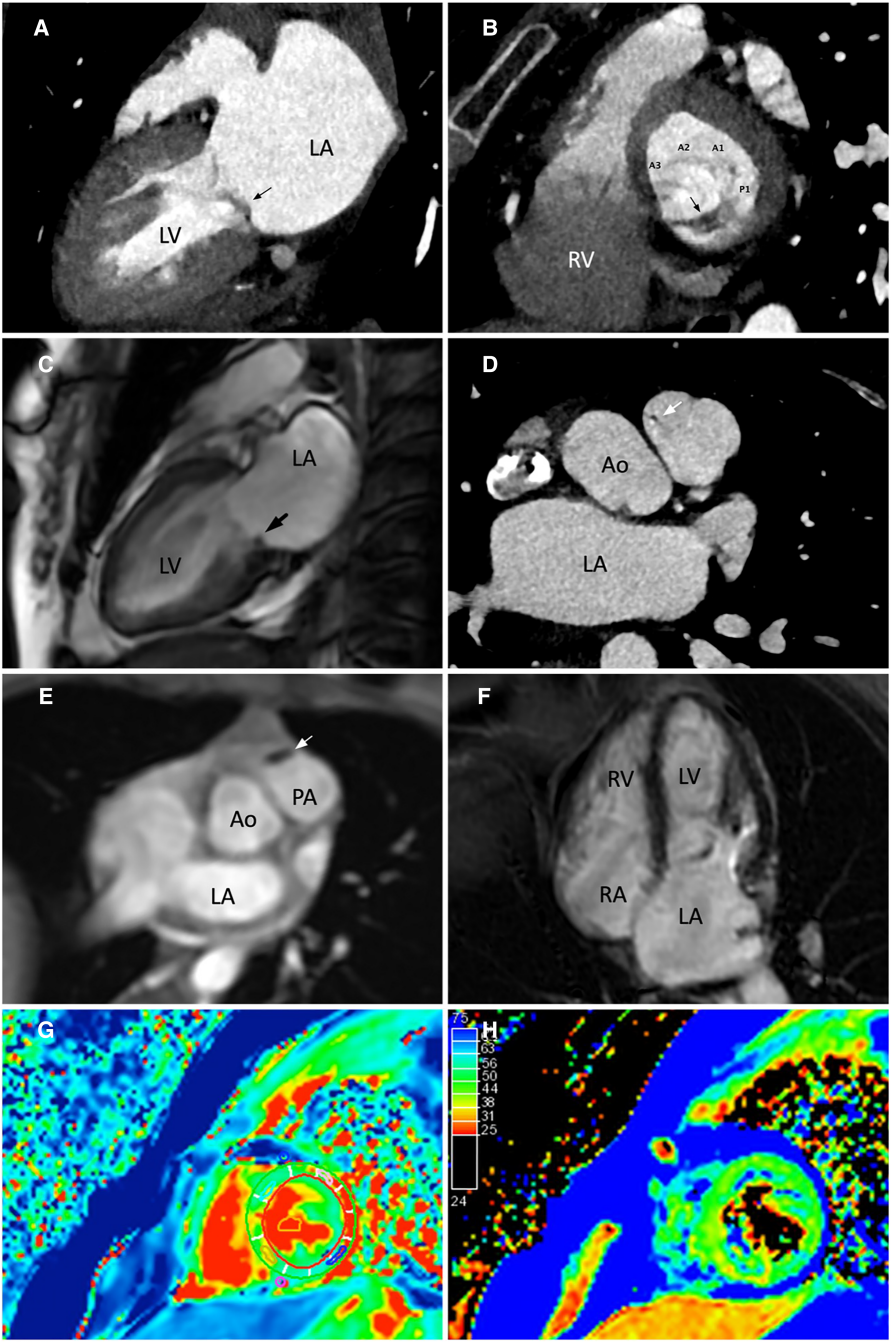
Evaluation of the mitral and pulmonary valve vegetations using cardiac computed tomography (CT) and cardiac magnetic resonance (CMR). (A,C) CT 2-chamber view and cine-CMR still image in end-systole showing a non-homogeneous, isodense mass involving the posterior leaflet of the mitral valve. (B) CT short axis view of the mitral valve from a basal short axis slice demonstrated the attachment of the vegetation to the P2 and P3 portions of the posterior mitral leaflet. The mitral valve was seen from a left ventricular perspective. (D,E) Transverse CT and CMR images through the right ventricular outflow tract showed a small oscillating mass on the anterior cusp of the pulmonary valve. (F) The myocardium showed no late gadolinium enhancement. (G,H) Normal findings on native-T1 and T2-weighted mapping images. Annotations: LA, left atrium; LV, left ventricle; RA, right atrium; RV, right ventricle; Ao, Aorta.
